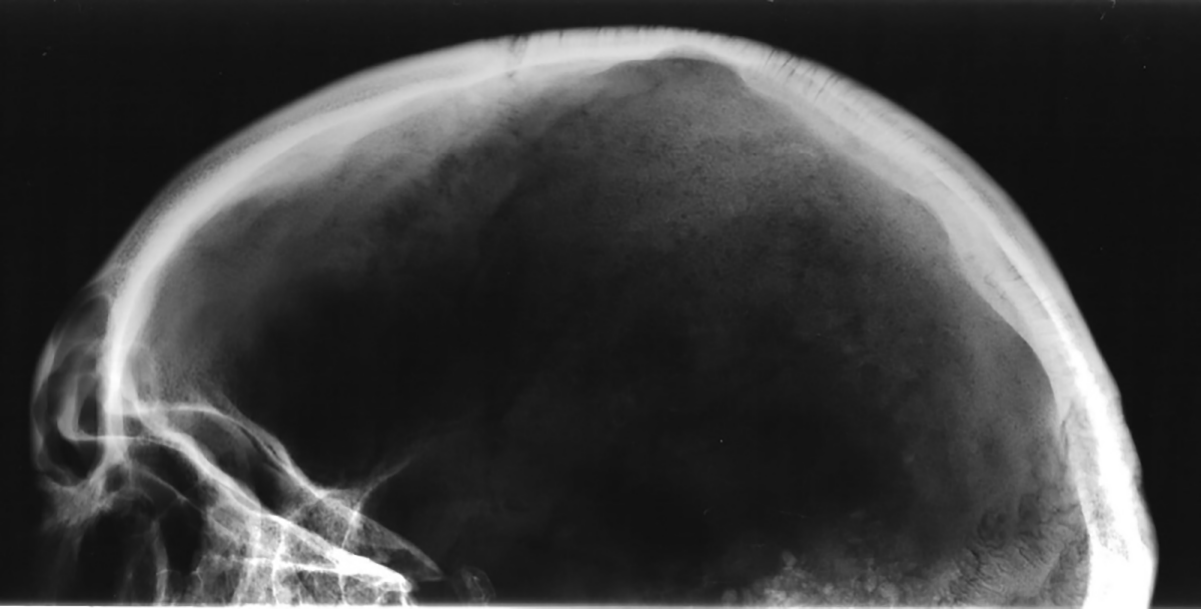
Left lateral view of the radiographed cranium of SK196 showing the characteristic “hair on end” pattern seen in porotic hyperostosis.
